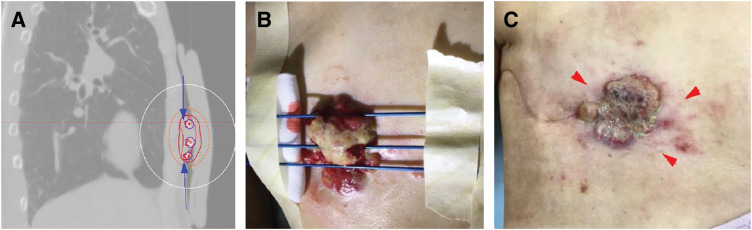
Brachytherapy delivered to the site of local chest-wall recurrence. (A) Treatment planning CT images for brachytherapy. (B) Intraoperative view of brachytherapy needle placement. (C) State of chest wall after 30Gy/6fr irradiations, with considerable tumor shrinkage. The tumor has shrunk within the arrowheads.
